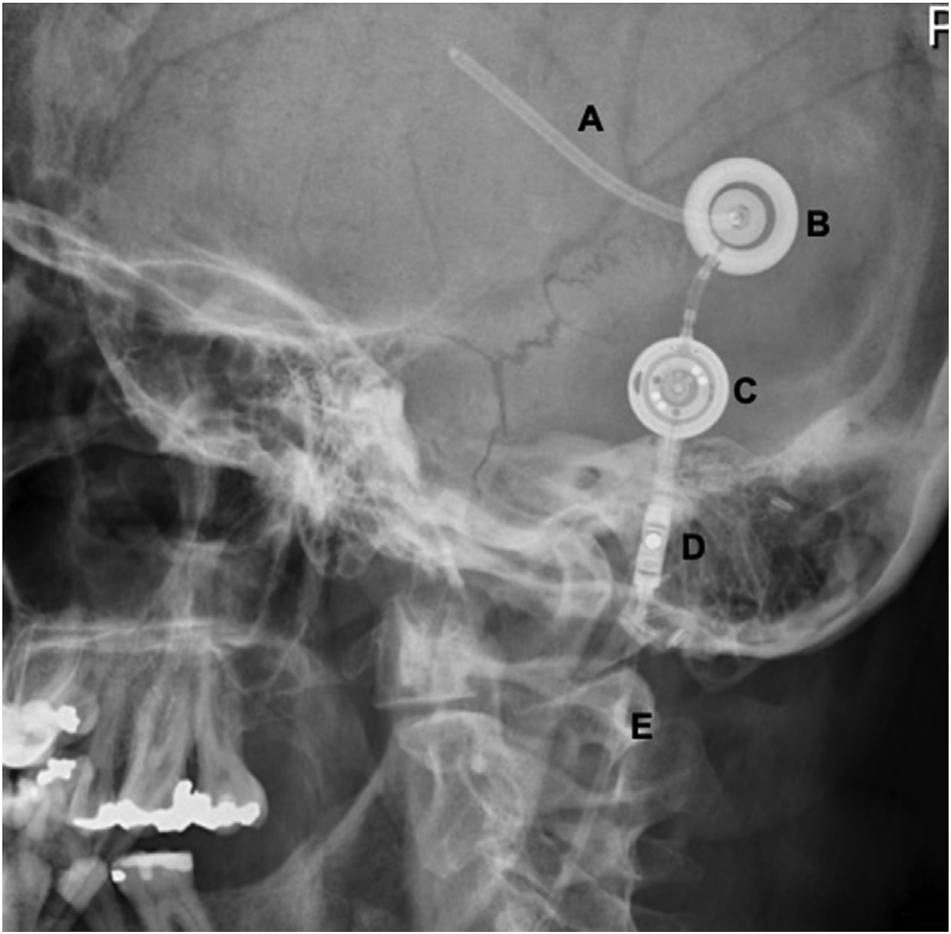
Lateral skull X-ray confirming a ventricular shunt including the proximal ventricular catheter (A), reservoir (B), adjustable valve (C), gravitation unit (D), and the distal catheter tunnelled subcutaneously (E).
